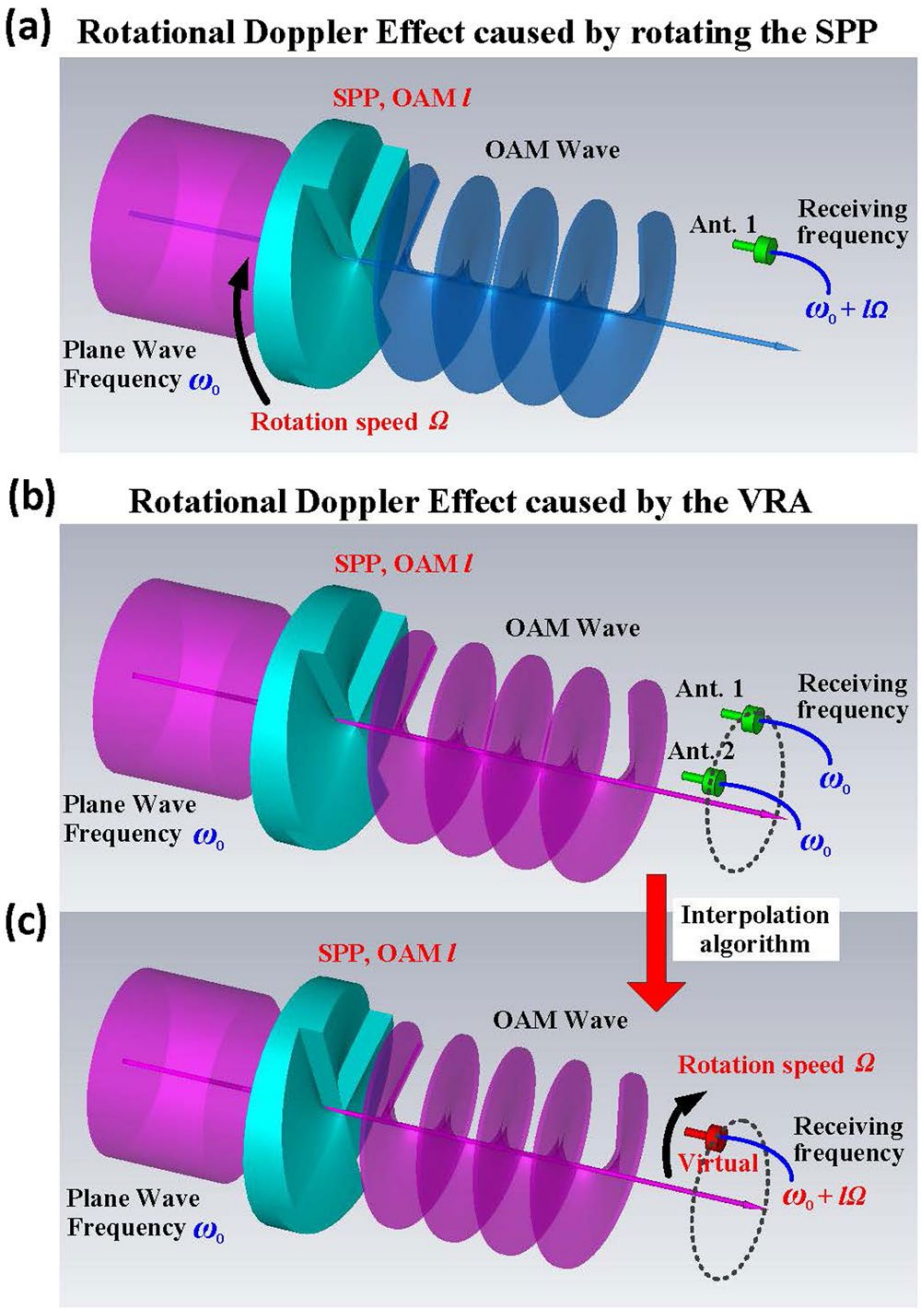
Doppler Effect caused by the VRA. (a) Detecting the OAM mode by rotating the SPP at the transmitter. Rotating the SPP of mode *l* with speed *Ω*, a frequency shift *l*
